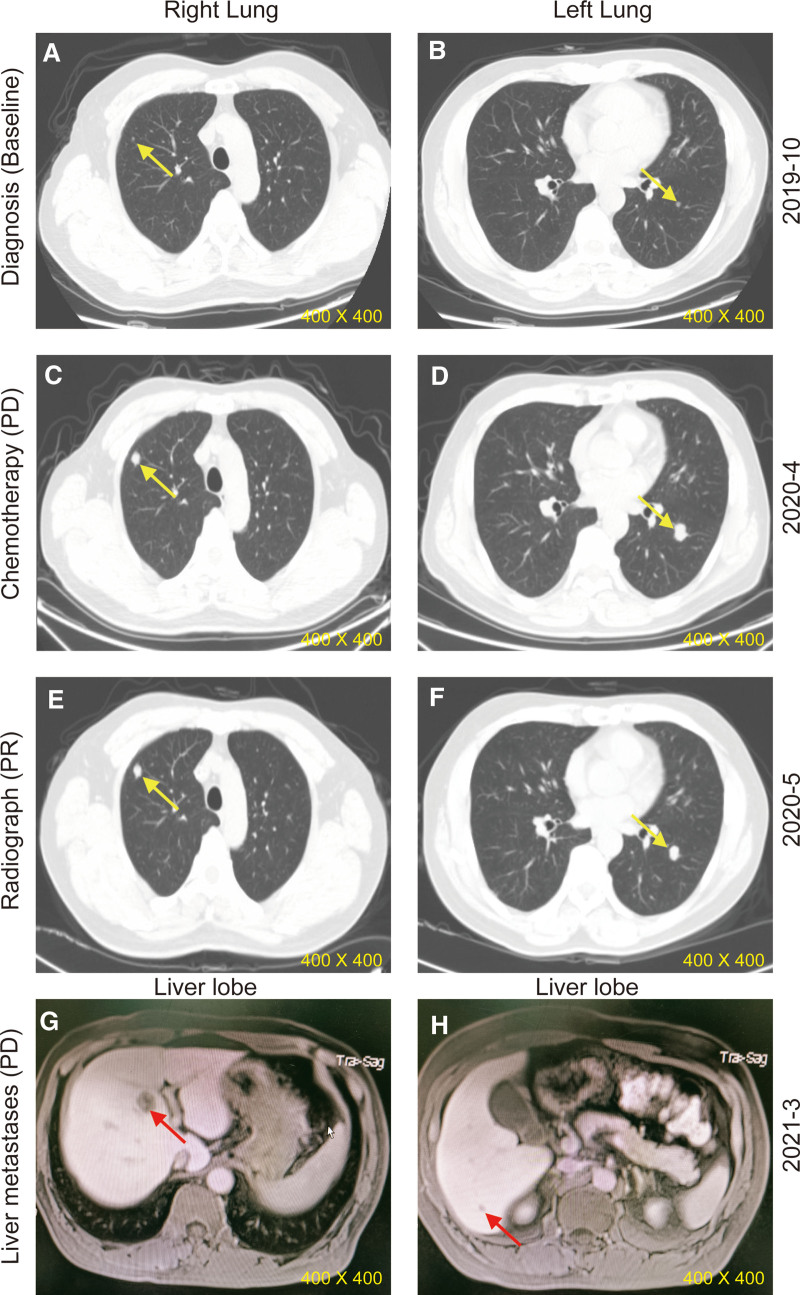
Image from chest computed tomography. (A) The apical part of the upper lobe of the right lung during diagnosis in October 2019; (B) the dorsal segment of the lower lobe of the left lung during diagnosis in October 2019; (C) the apical part of the upper lobe of the right lung during disease progression in April 2020; (D) the dorsal segment of the lower lobe of the left lung during disease progression in April 2020; (E) the apical part of the upper lobe of the right lung with PR after radiofrequency ablation in May 2020; (F) the dorsal segment of the lower lobe of the left lung with PR after radiofrequency ablation in May 2020; liver metastases on coronal (G) and axial (H) MRI images in March 2021. PR = partial response.
